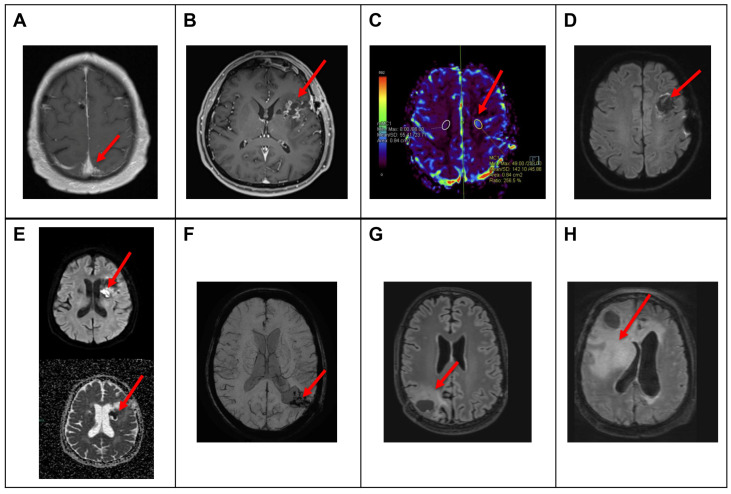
Representative post-treatment MRI features evaluated in the study cohort. Red arrows in all panels indicate the imaging feature described in the corresponding panel label. Note: (A) Postoperative residual tumor adjacent to the resection cavity on contrast-enhanced T1-weighted imaging. (B) Residual tumor showing contrast enhancement along the surgical cavity wall. (C) Increased relative cerebral blood volume (rCBV) on perfusion imaging. (D) Diffusion restriction within tumor tissue on diffusion-weighted imaging. (E) Postoperative infarction adjacent to the surgical cavity. (F) Postoperative hemorrhage visible on susceptibility-weighted imaging. (G) Peritumoral edema on FLAIR imaging. (H) Midline shift secondary to postoperative mass effect.
